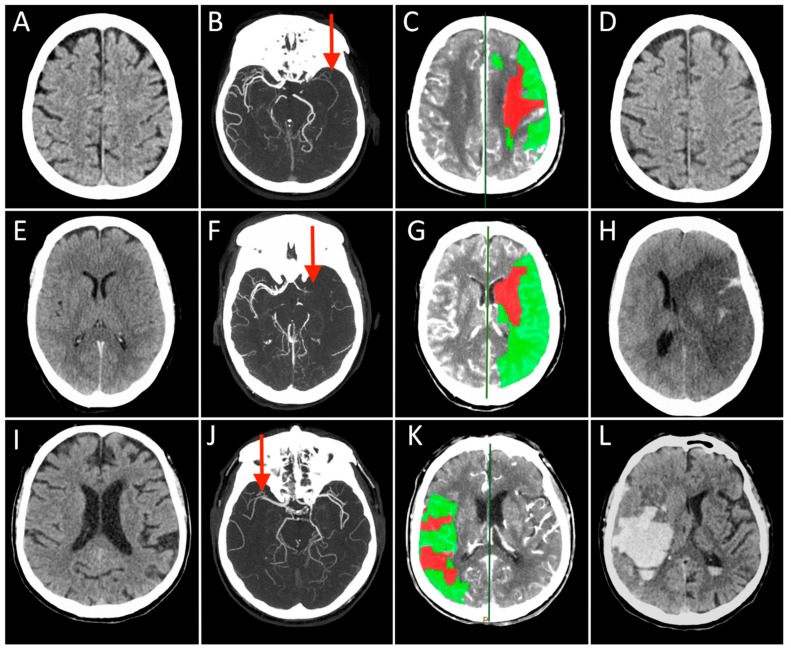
Each row represents a single patient’s images derived in different modalities and timepoints (Case 1 presented in (A–D), Case 2 in (E–H), and Case 3 in (I–L)). The first three columns on the left show the Non-Contrast-CT (NCCT), multiphase CT Angiography (CTA), and CT Perfusion (CTP) performed at hospital arrival, while the last column on the right displays the NCCT acquired at 24 h after stroke. On presenting NCCT (A,E,I) no early ischemic changes can be seen in the brain tissue in Case 2 and 3 (ASPECT score = 10), while a tissue swelling was detected in the right insular lobe in Case 1 (ASPECT score = 9, not shown). The mCTA images identified proximal (M1 segment) Middle Cerebral Artery (MCA) occlusion in the left hemisphere in (B,F), and contralateral side in (J) (red arrows). CTP showed in all cases a small infarct core corresponding to CBV lesion (red) and a large ischemic penumbra consisting of the difference between MTT and CBV lesions (green), representing the expected “salvageable” tissue after recanalization. In these patients, CBV lesion volume ≤ 50% of MTT lesion size. All patients were treated with combined IVT and MT, obtaining a complete recanalization, but the 24 h NCCT showed three different conditions: no ischemic lesion visible (D), complete MCA territory infarct associated with a massive cerebral oedema (H), and a vast hemorrhagic transformation of the ischemic lesion.
